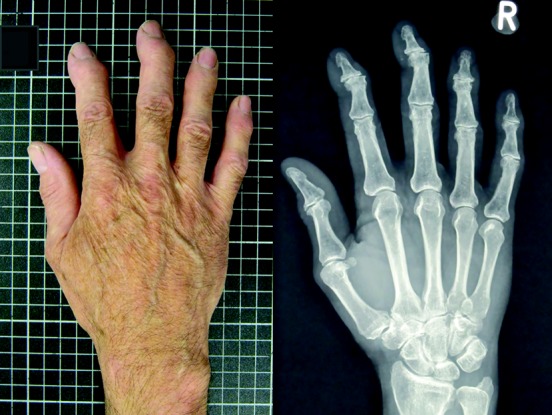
In this study, the associations of photographic hand osteoarthritis (OA) with radiographic OA and clinical features were examined to explore the construct validity of hand photography as an indicator of hand OA. An example of a hand photograph and its corresponding radiographic image is shown.
